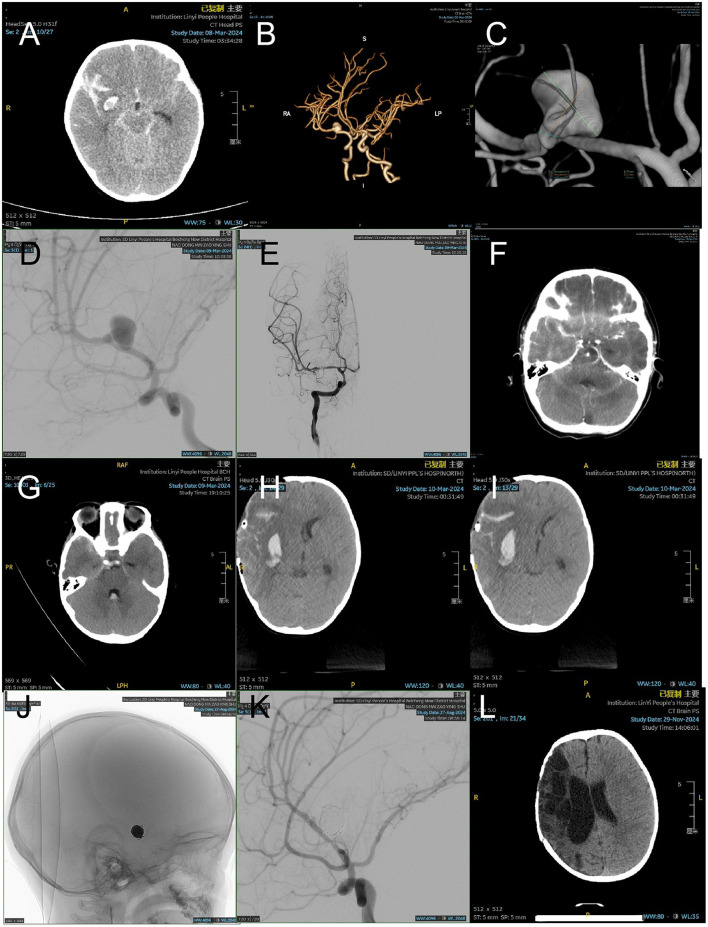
Imaging of case 1. (A, B) Initial cranial CT angiography (CTA) on admission demonstrating a right middle cerebral artery (MCA) aneurysm with subarachnoid hemorrhage. (C, D) Cerebral digital subtraction angiography (DSA) showing an aneurysm located in the M1 segment of the right MCA. (E) Immediate post-embolization angiography demonstrating dense packing of the aneurysm. (F) Immediate post-procedural CT following intracranial aneurysm embolization. (G) CT scan obtained after sudden onset of pupillary dilation. (H, I) CT scans following decompressive craniectomy. (J, K) Follow-up DSA demonstrating complete obliteration of the aneurysm, with mild proximal stenosis of the parent artery. (L) CT scan after cranioplasty.
